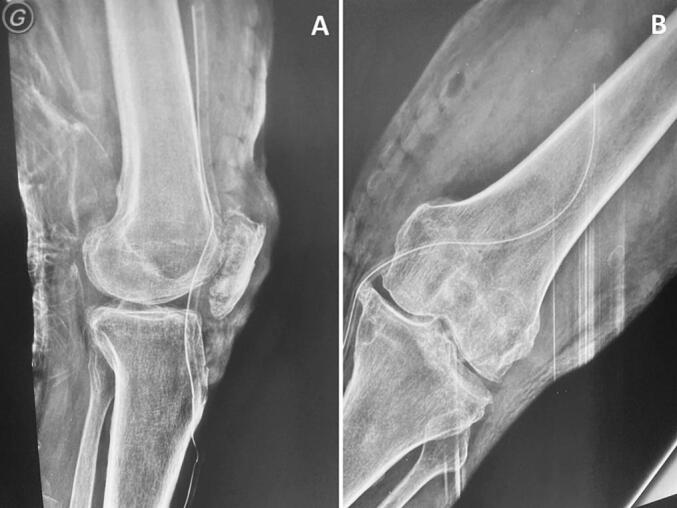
(A + B) Postoperative radiographs of the left knee joint showing the successful excision of the mass with moderate Ahlback stage 2 osteoarthritis.
